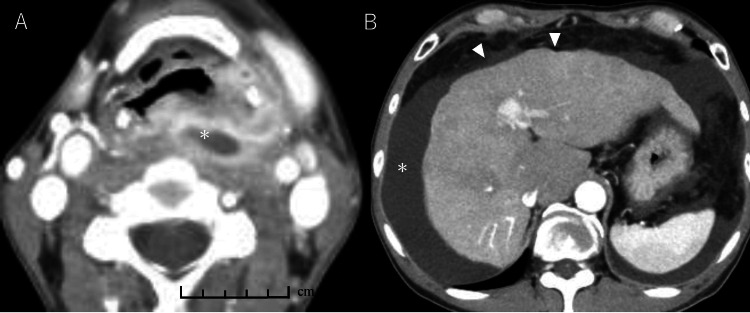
Contrast-enhanced CT of the neck and abdomen. (A) In the axial view of a contrast-enhanced computed tomography (CT) scan of the neck, a tumorous lesion (asterisk) is observed, primarily located in the left piriform recess. The lesion has partially extended beyond the thyrohyoid membrane, infiltrating the extralaryngeal space. (B) The contrast-enhanced CT scan of the abdomen reveals an irregular hepatic surface (arrowhead) and a substantial presence of ascites (asterisk).
